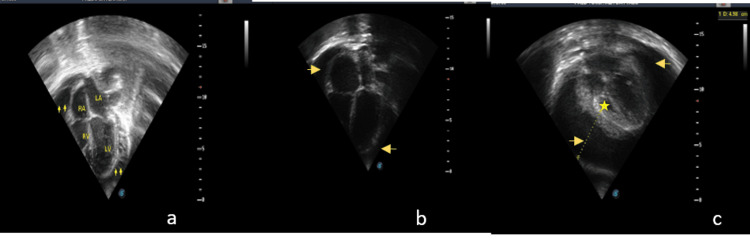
Echocardiography of three patients showing (a) apical 4-chamber view with mild circumferential effusion with fibrin strands (arrows), (b) apical 4-chamber view with moderate clear effusion (arrows), (c) sub-xiphoid view with severe effusion and fibrin deposits, thickened pericardium (arrows), and collapse of right ventricle (star)
